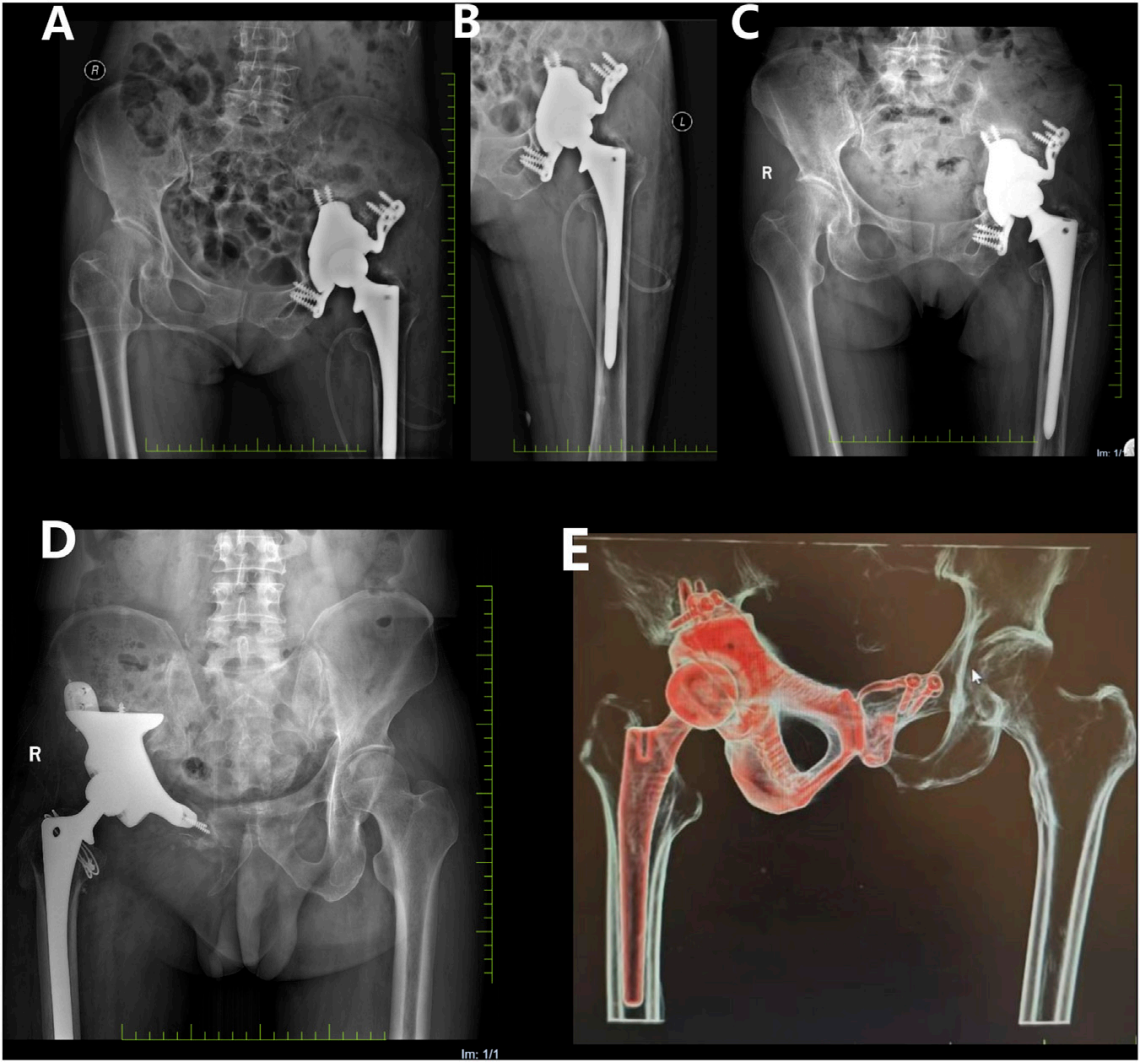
Postoperative X-ray films. (A,B) on the first day after operation, the prosthesis position of patient 1 was satisfactory, and there was no sign of displacement or loosening. (C) 18 months after operation, compared with the previous X-ray film, the prosthesis position of patient 1 remained satisfactory without displacement; (D) At 18 months after operation, the position of prosthesis of patient 3 remained satisfactory without displacement; (E) 18 months after operation, the prosthesis position of patient 2 remained satisfactory without displacement.
